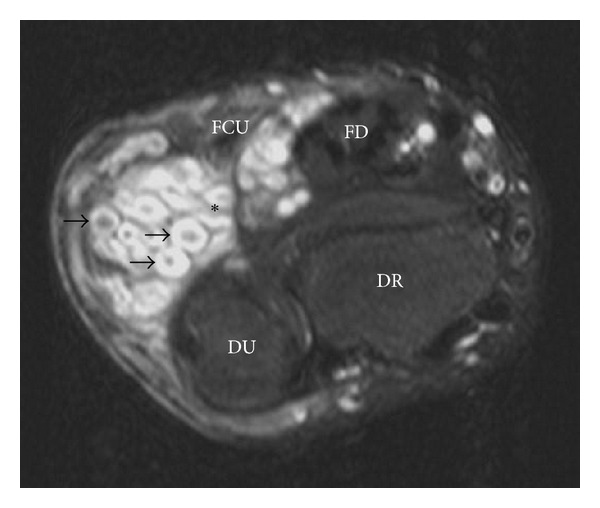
Axial T2-weighted wrist MRI with fat suppression—multilobulated right wrist mass (anterior/ulnar aspect). Individual lesions (arrows) show target signs: rim of high-signal intensity surrounding central low-signal intensity. *usual location of ulnar nerve. DR: distal radius; DU: distal ulna; FD: flexor digitorum longus; FCU: flexor carpi ulnaris.
